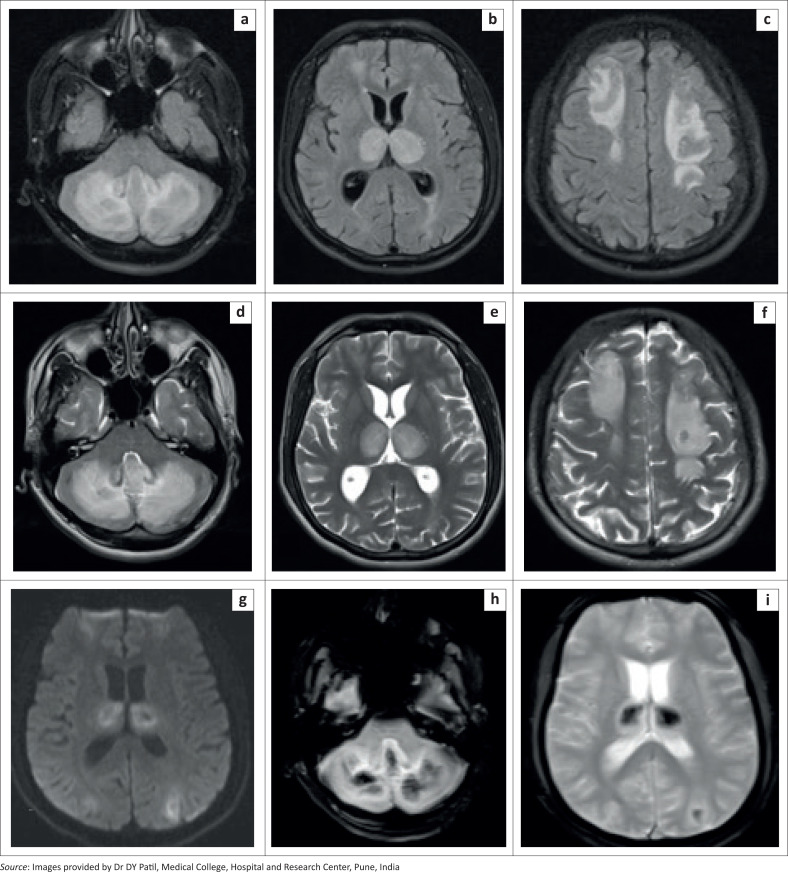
[Case 1]: Brain MRI in a 25-year-old male diagnosed with dengue infection indicates symmetric FLAIR (a-c) and T2 hyperintense areas of altered signal intensity in the vermis, bilateral cerebellar hemispheres, thalami and bifrontal and biparietal white matter (d-f) with patchy diffusion restriction at DWI (g) – ADC not presented. Areas of blooming on GRE images (h, i), representing haemorrhages, are seen.
